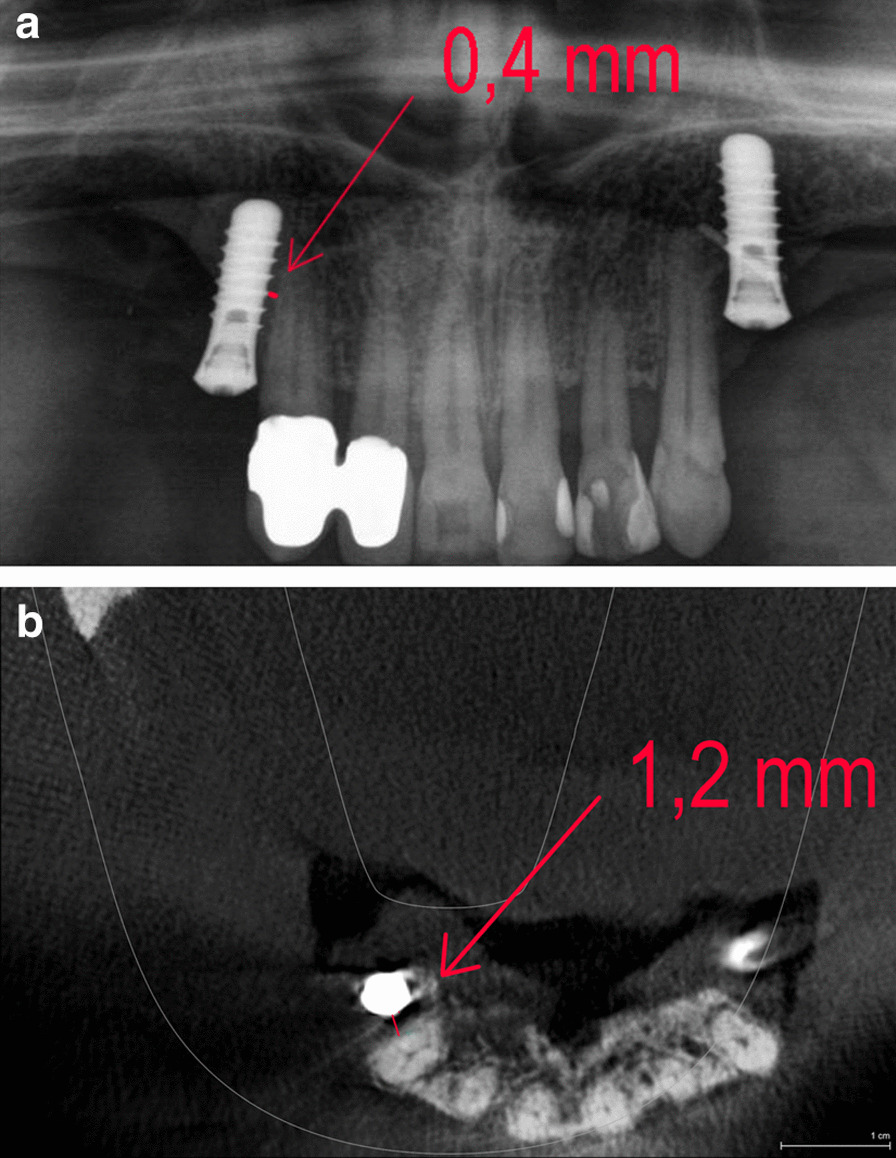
IRD measuring technique on PAN (a) and CBCT (b) without overlapping structures in PAN. a Outer surfaces of implant and neighboring root are assessed on PAN images. At the area of smallest distance between implant and adjacent root surface the local implant thread was used as reference point for IRD measurement (red line). b Outer surfaces of neighboring implant and root were assessed at the level of the reference thread on axial CBCT images. IRD represents distance between implant and adjacent root surface at the level of the reference thread (red line)
